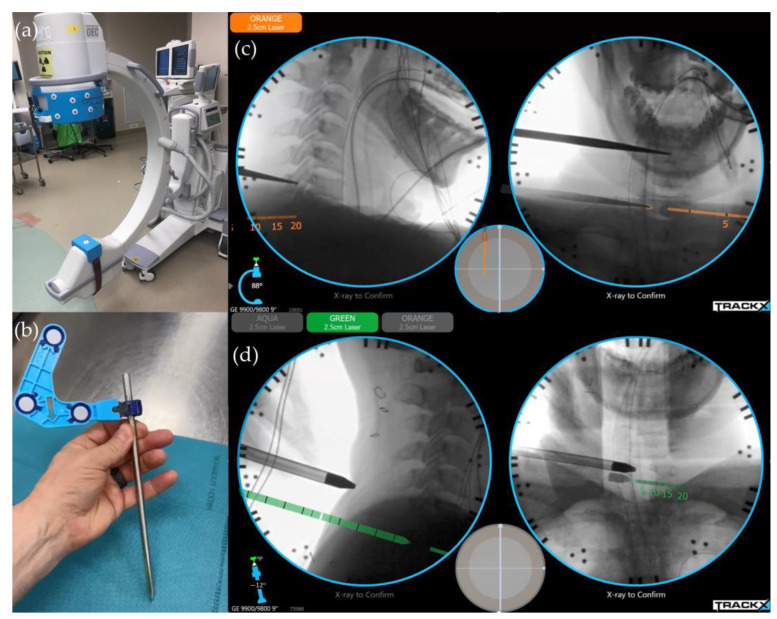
The setup and operation of a real-time surgical instrument tracking system. (a) Fiducials are attached to a conventional C-arm, which does not require affixation to the patient or operating table. (b) Trackers are secured onto the surgical instruments using quick-connect clamps for efficient attachment. (c,d) With the instrument tracking system, the surgeon can observe the instrument’s position and trajectory in real time, as the orange and green indicators displayed in both anteroposterior and lateral radiographic views. This feature aids in (c) precision skin incision planning and (d) ensures accurate dilator placement on the facet joint.
